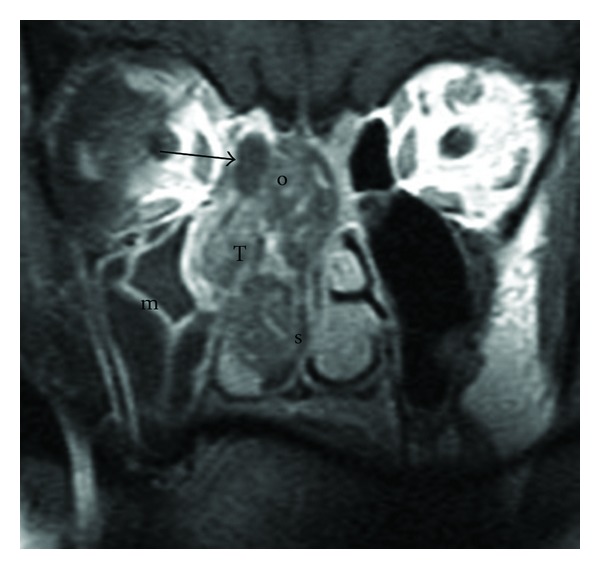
Initial lesion, MRI: MRI confirms CT scan images (T: Tumor, o: olfactory cleft, *→*: ethmoidal attic, and m: maxillary sinus). There is no typical signal of chordoma (T1 hyposignal and T2 very intense hypersignal).
